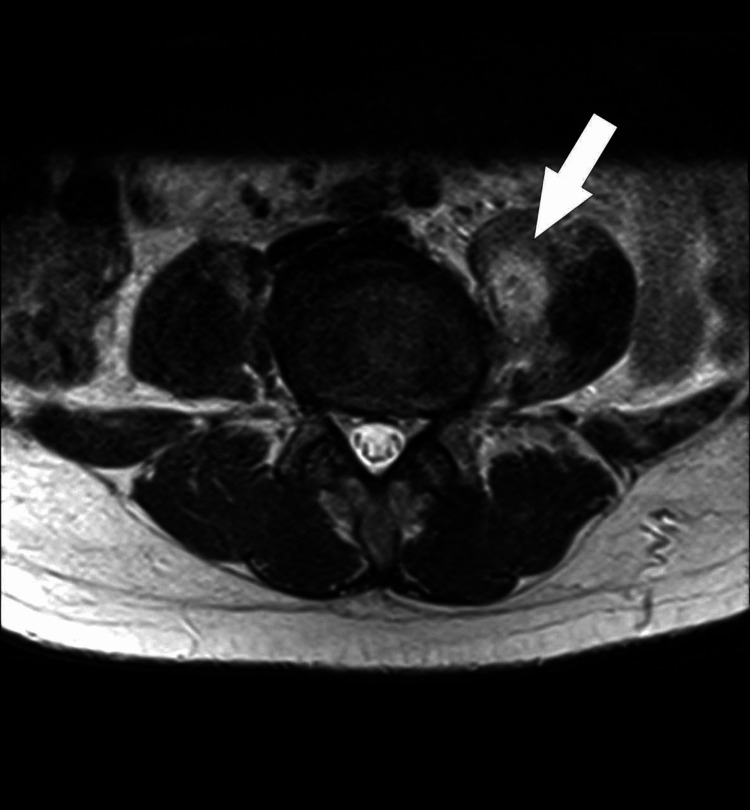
MRI axial T2-weighted sequence of the lower abdomen which reveals a small abscess within the left psoas (arrow), with adjacent muscular edema. There is also minimal muscular edema within the right psoas muscle. MRI: magnetic resonance imaging
